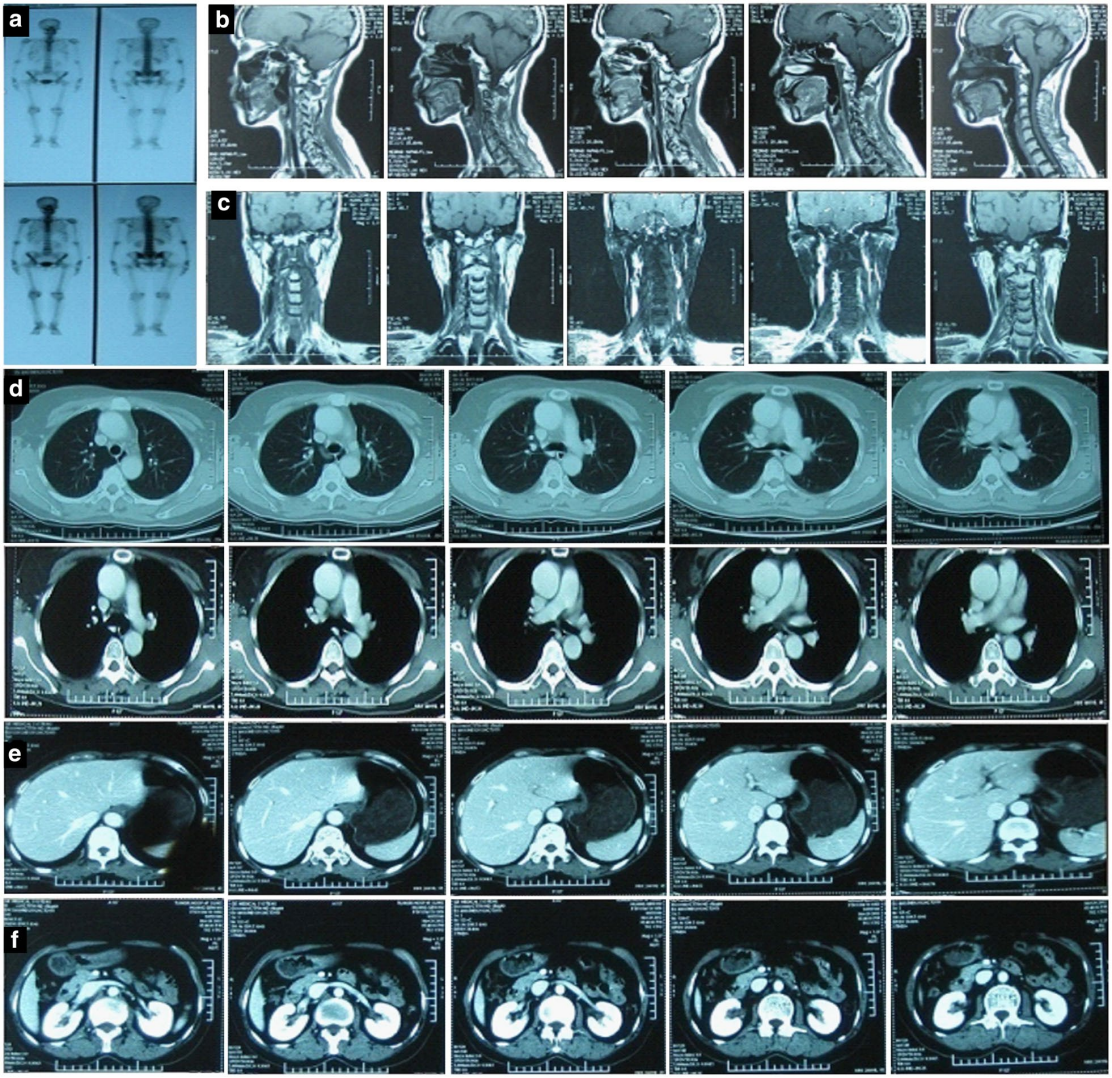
Examination of an NPC patient 20 years after 50 Gy radiotherapy plus HPD. a whole body bone scan image without abnormally increased uptake; b nasopharyngeal neoplasm disappears on nasopharyngeal magnetic resonance image (MRI); c cervical lymph node metastases are not found on cervical MRI; d chest computed tomography (CT) is normal; e upper abdominal CT without any abnormal lesion; f metastases are not found on lower abdominal CT
